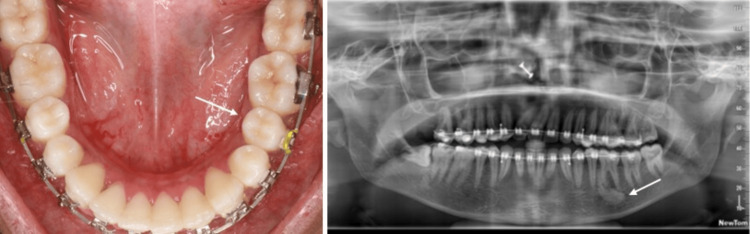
A 21-year-old female presented with malocclusion (A) Intra-oral view shows no change in the clinical appearance of the lesion 11 months after initiation of treatment (arrow). (B) 11-month follow-up OPG reveals no changes in the radiographic appearance of the lesion (arrow). OPG, orthopantomograph
